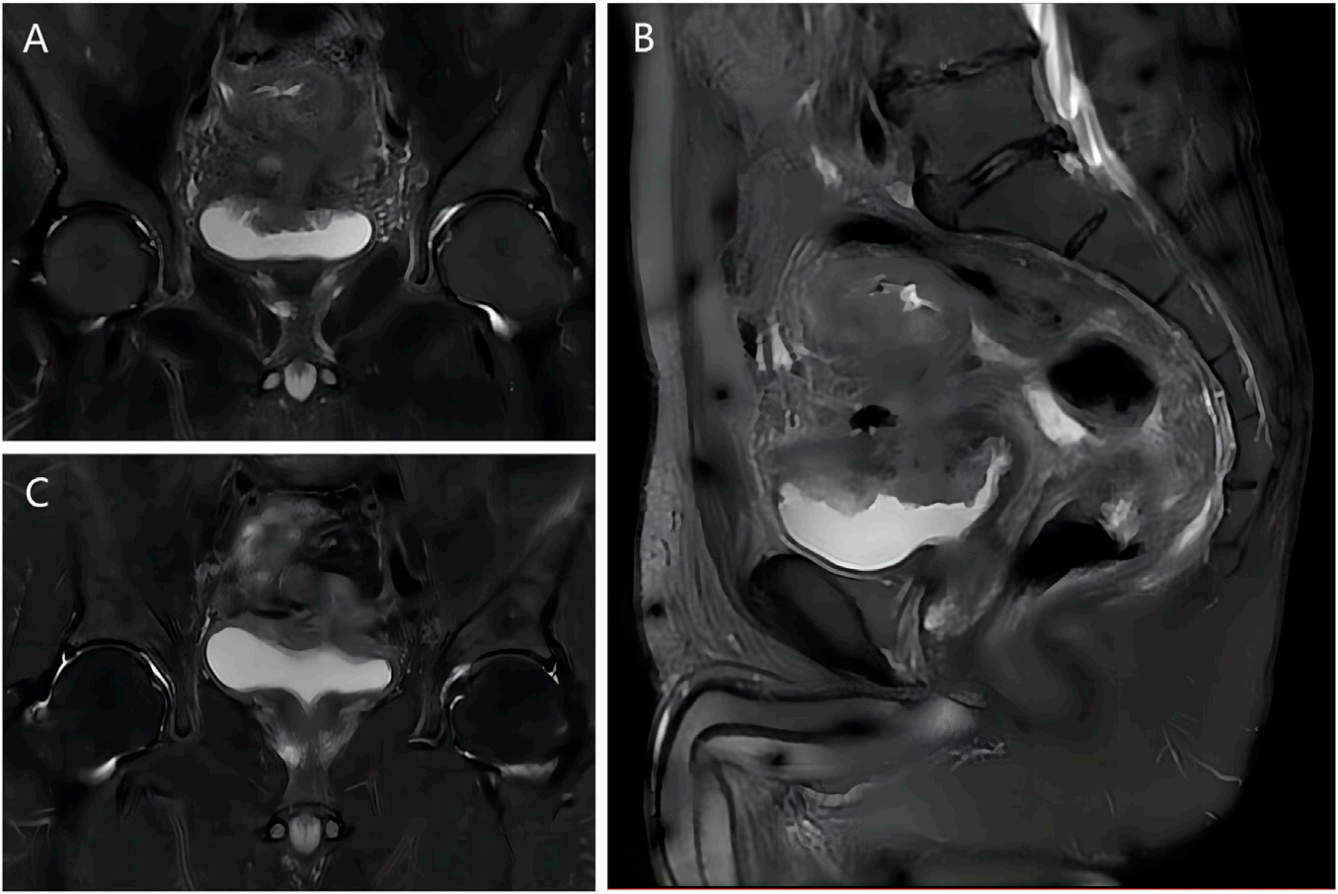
MRI images of patient before and after NAC. (A) T2 coronal image before NAC; (B) T2 sagittal image before NAC; (C) T2 coronal image after NAC.
